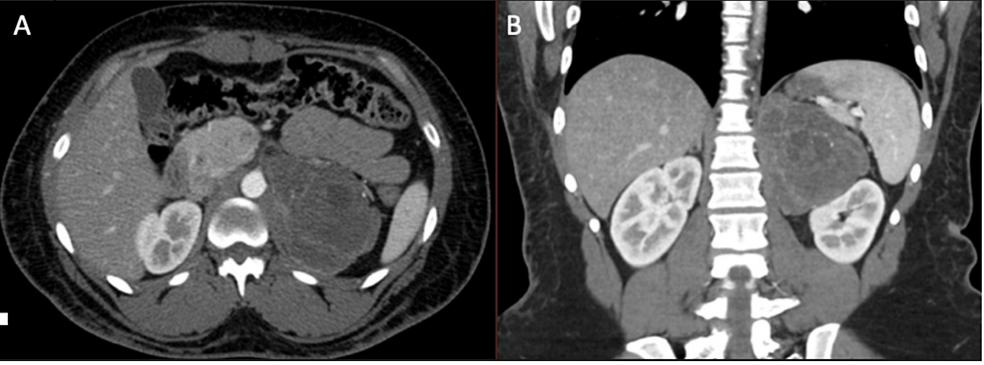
Axial (A) and coronal (B) contrast-enhanced CT images show a large left retroperitoneal mass with multiloculated cystic and necrotic components, thin enhancing walls/septa, and isolated punctate calcifications. There is a mass effect on the upper pole of the left kidney.
